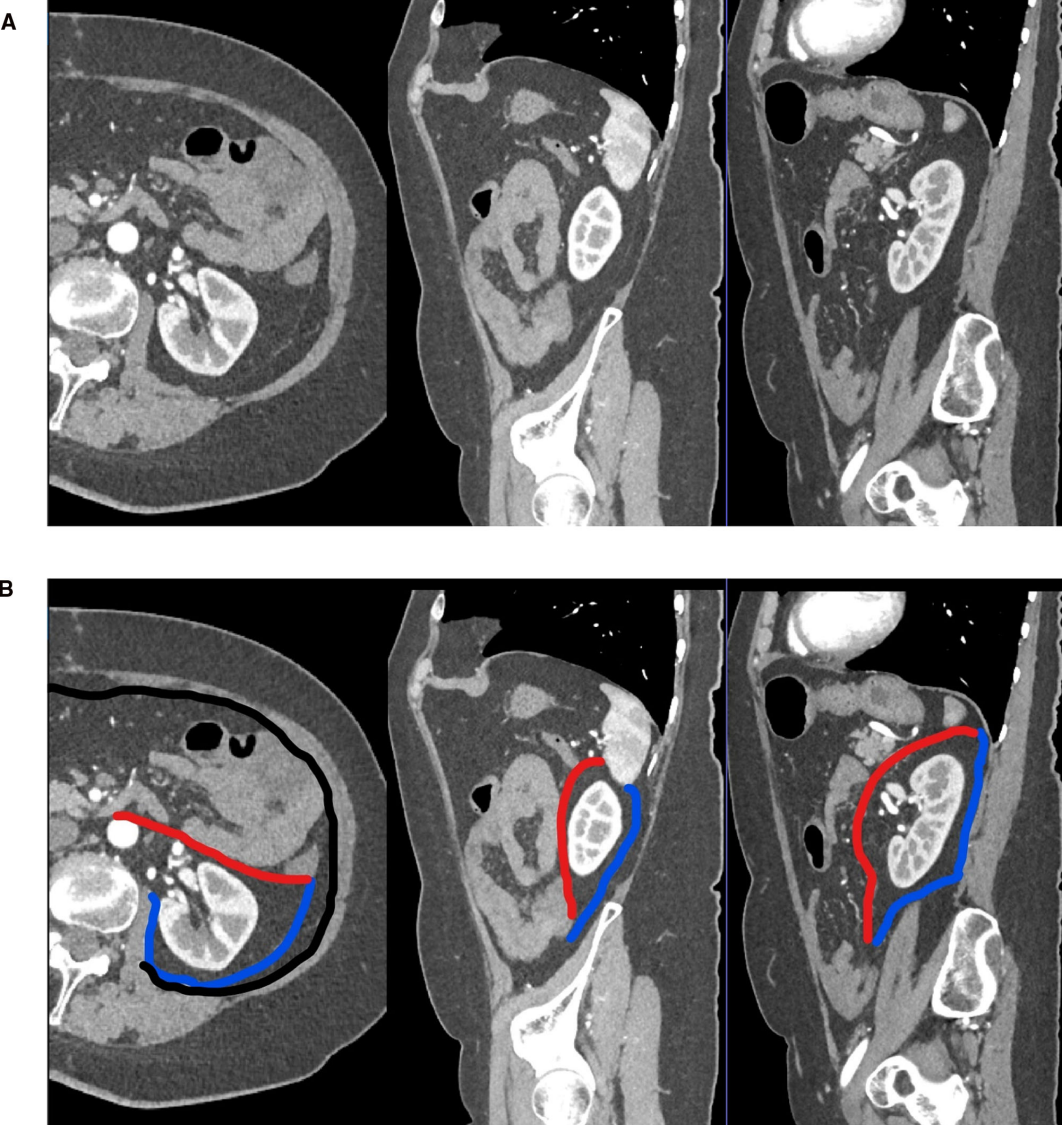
Computed tomography imaging with a reconstruction of the transverse and sagittal planes with contrast enhancement (A) and emphasizing the perirenal fat pad and its limits. (B) Same images with the posterior renal fascia highlighted in blue, the anterior renal fascia in red, and TF in black.
